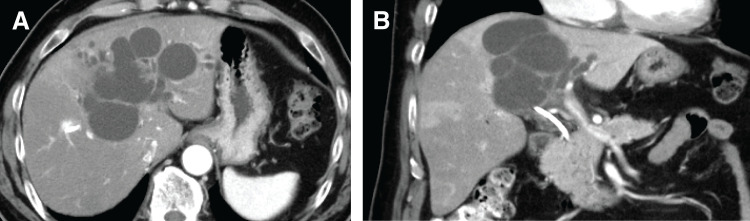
Enhanced CT and ERCP of the case (on admission to our hospital). (A, B) Enhanced CT showing a lesion protruding from the medial bile duct into the left hepatic duct, along with a dilated intrahepatic bile duct. ERCP, endoscopic retrograde cholangiopancreatography
